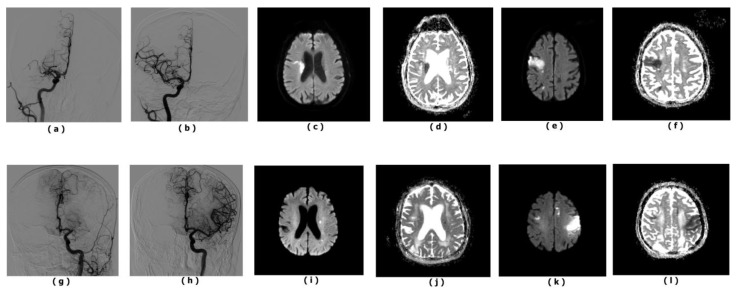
Representative cases of new DWI lesions after complete (TICI 3) reperfusion following mechanical thrombectomy. (a) Case 1 pre-thrombectomy digital subtraction angiography (DSA) demonstrating occlusion of the right middle cerebral artery (MCA) M1 segment. (b) Post-thrombectomy DSA showing complete reperfusion (TICI 3) with full restoration of distal flow. (c,d) Baseline diffusion-weighted imaging (DWI) and corresponding apparent diffusion coefficient (ADC) maps showing the initial infarct core. (e,f) Follow-up DWI and ADC images obtained within 48 h demonstrating newly developed ipsilateral frontal and parietal lobe cortical diffusion-restricted lesions, spatially distinct from the baseline infarct. (g) Case 2 pre-thrombectomy DSA demonstrating MCA M1 segment occlusion. (h) Post-thrombectomy DSA showing complete reperfusion (TICI 3). (i,j) Baseline DWI and ADC images showing small initial foci. (k,l) Follow-up DWI and ADC images demonstrating new diffusion-restricted lesions, including ipsilateral and contralateral foci. All new lesions were confirmed by corresponding low signal on ADC maps to exclude T2 shine-through. Follow-up MRI was performed within 48 h after the procedure.
